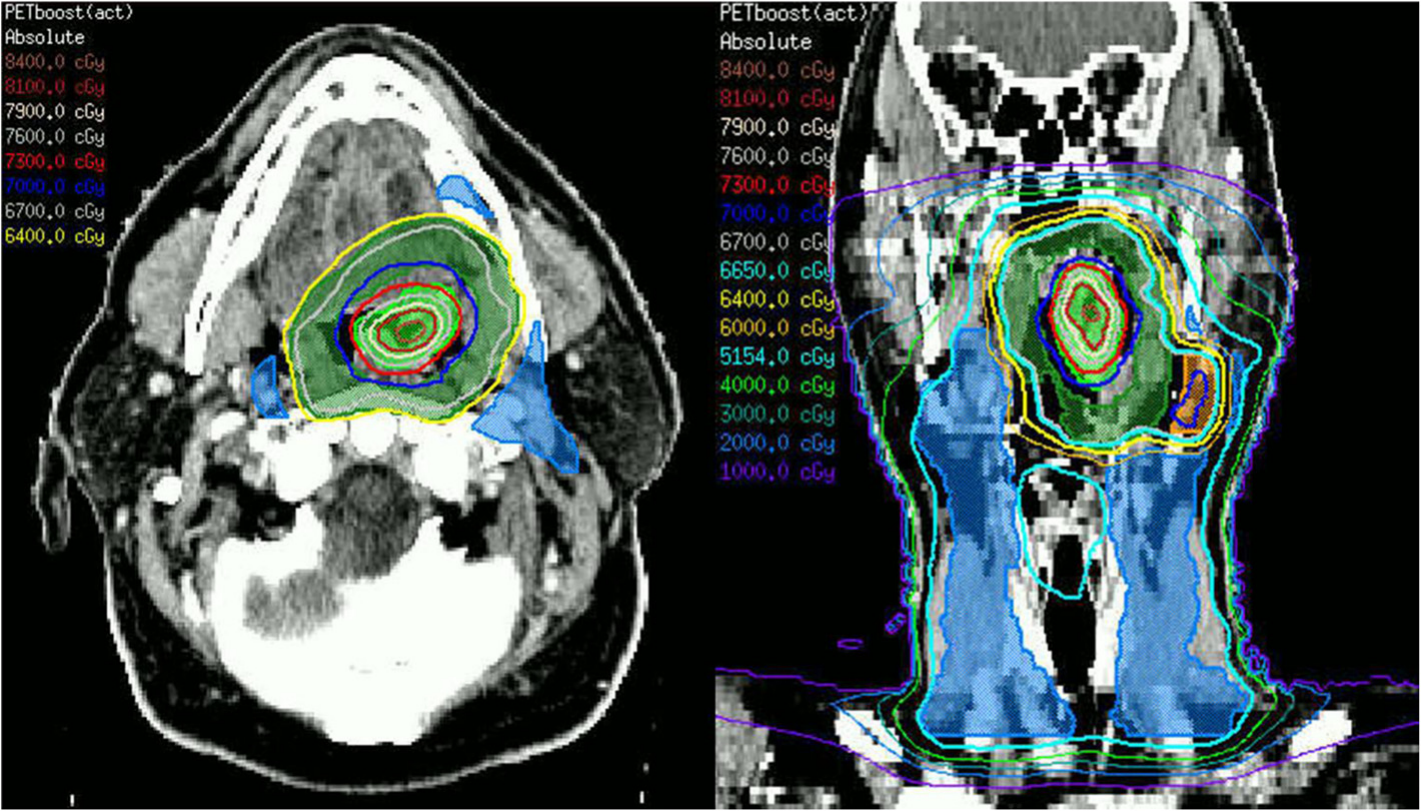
Dose in the redistributed radiotherapy regimen. Transverse (left) and coronal (right) view with isodose lines. In the dose-painted redistriburtion radiotherapy regimen, the GTV-FDG-PET is defined by an automatic iso-contour at 50% of the maximum uptake in the primary tumour. This is expanded by 3mm to form the PTV-FDG-PET. The GTV-primary should at least encompass the GTV-FDG-PET and is expanded by 10mm to form the PTV-primary. The PTV-FDG-PET will receive 35 fractions to a maximum total dose of 84 Gy to 2% of the volume (PTV-FDG-PET), a minimum dose of 70 Gy and a mean dose of approximately 77 Gy. The PTV-primary outside the PTV-FDG-PET will receive 35 fractions to a total mean dose of 67 Gy (ranging from 64-70 Gy).
